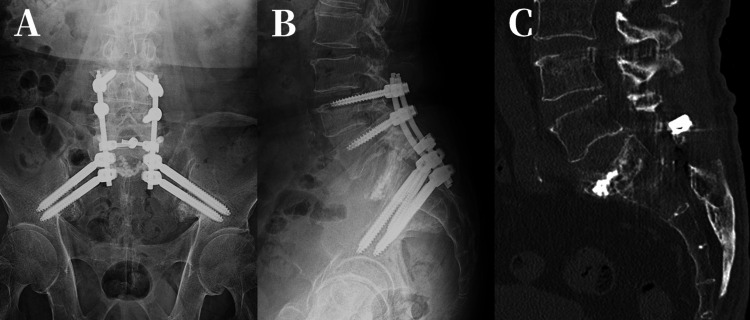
Anteroposterior, Lateral and Sagittal images. (A) Anteroposterior (B) Lateral (C) Sagittal CT image showing bone union (1 year after surgery). CT: computed tomography.
